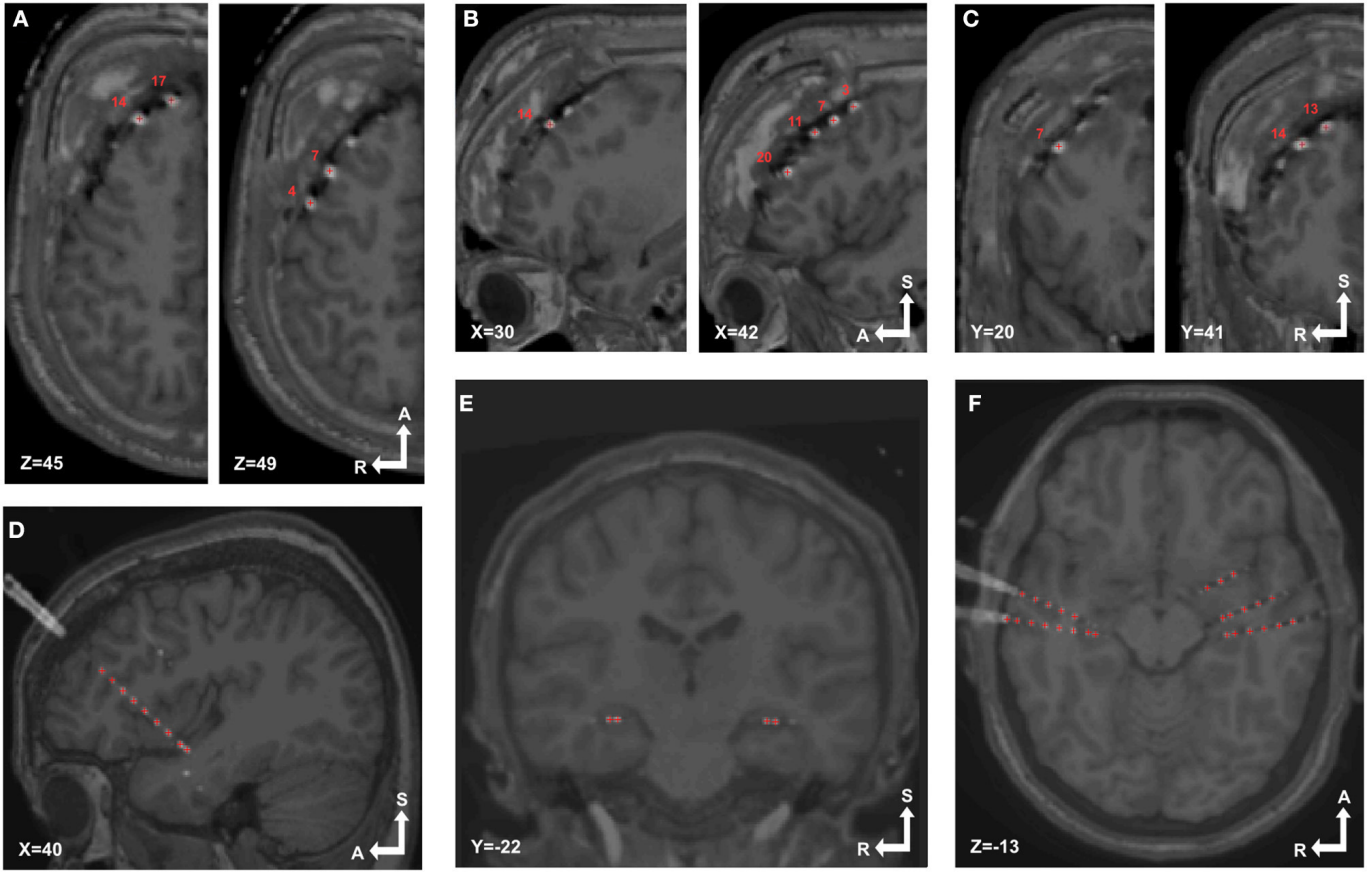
Localized electrodes in MRI-CT. MRI-CT blend images showing examples of electrode artifacts and detected coordinates (red crosses) superimposed. (A–C) Axial, sagittal and coronal views (respectively) of patient 6, implanted with a 4 × 5 grid over the right frontal lobe. (D) Sagittal view of patient 14 showing a depth electrode array in the insular cortex. (E,F) Coronal and sagittal view of patient 18 showing bilaterally implanted electrodes in hippocampi and temporal lobes.
